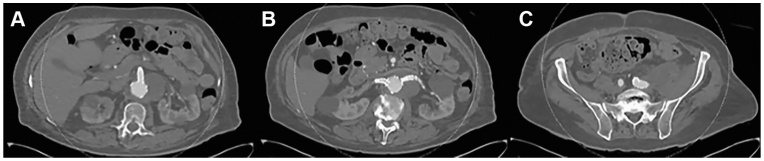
Representative axial images from 6-month follow-up computed tomography (CT) angiogram that demonstrates appropriate exclusion of the paravisceral penetrating aortic ulcer (PAU) and associated contained rupture (A) with patent superior mesenteric artery (SMA) (A) and bilateral renal arteries (B), as well as exclusion of the left common iliac ulcer and associated contained rupture (C).
